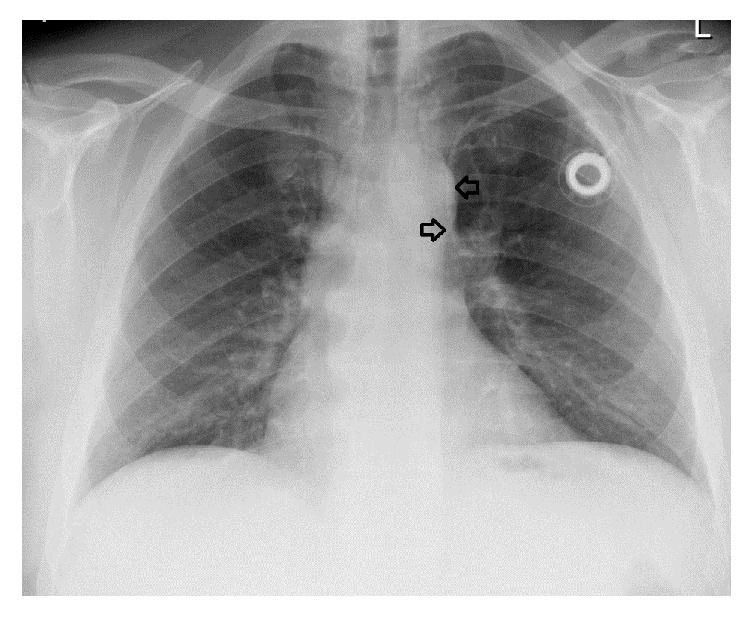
The a.p. chest X-ray shows the port catheter in the left-sided superior vena cava (black arrows).
